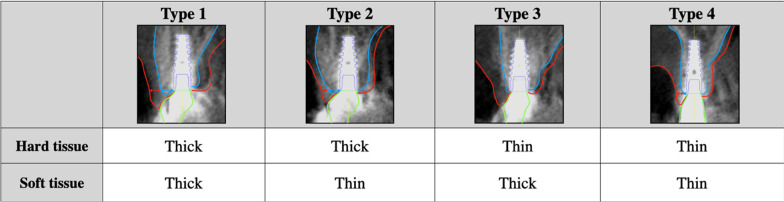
Representative CBCT images for classification of implants according to thickness of labial/buccal hard and soft tissue width. Cutoff thresholds were determined 1.6 mm for hard tissue, 2.5 mm for soft tissue. Implants with thick hard and soft tissue were classified as Type1, those with thick hard tissue and thin soft tissue were as Type 2, those with thin hard tissue and thick soft tissue were as Type 3, and those with thin hard and soft tissue were as Type 4
